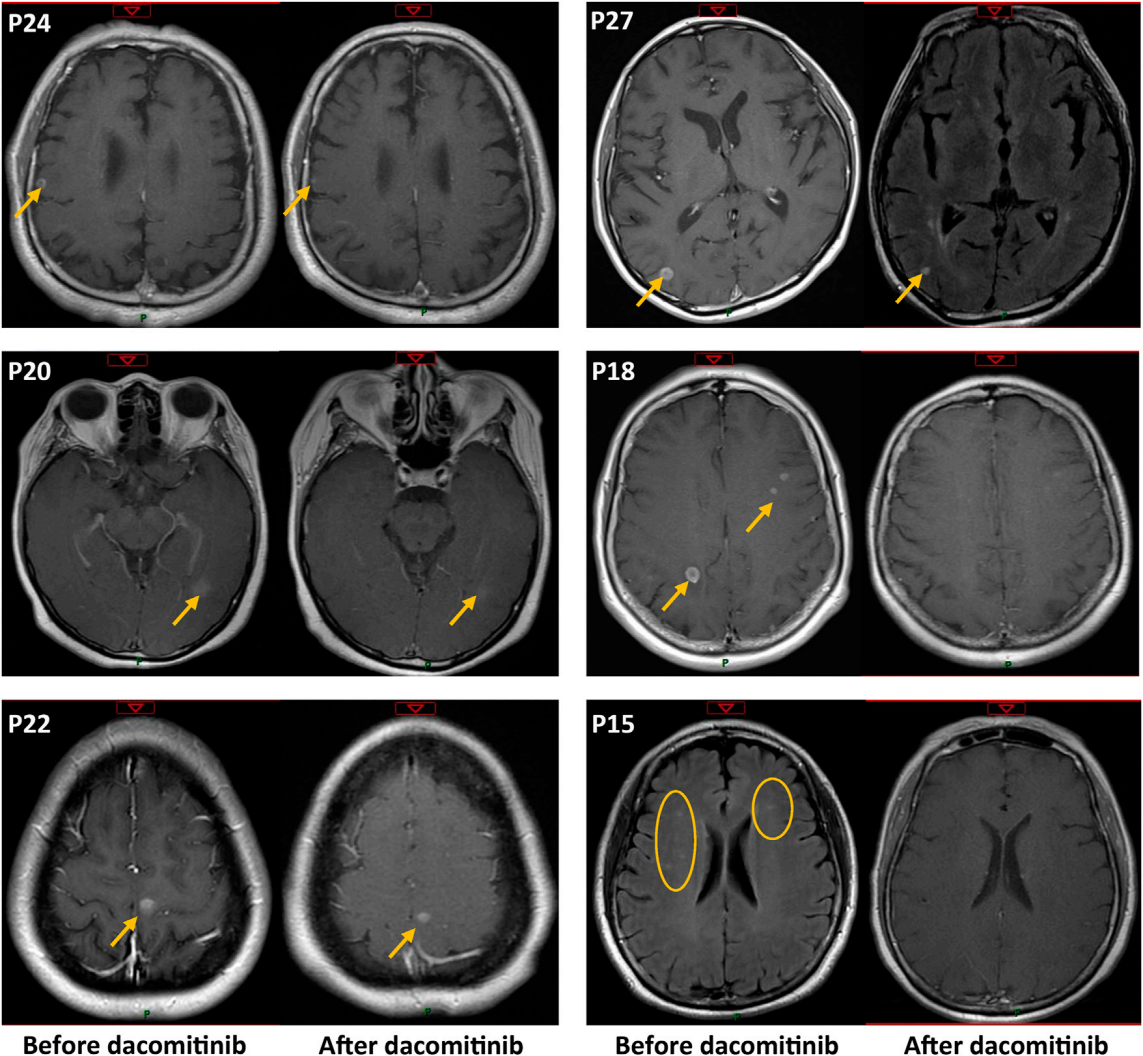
Enhanced MRI confirmed intracranial metastasis control before and after dacomitinib treatment in six representative patients. The patient number is consistent with Figure 1 but not Figure 2.
